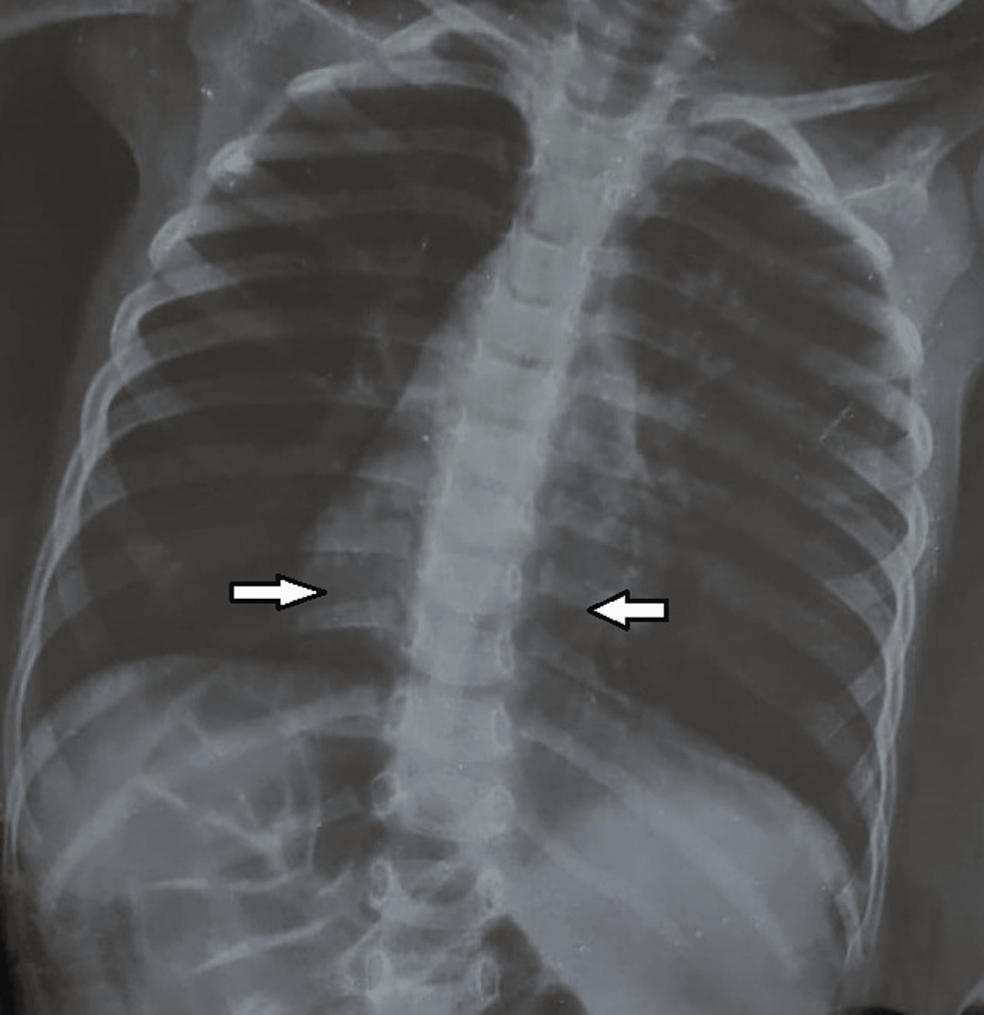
Chest X-ray showing dextrocardia (right arrow) and left upper lobar pneumonia with minimal homolateral pleural effusion (left arrow)
